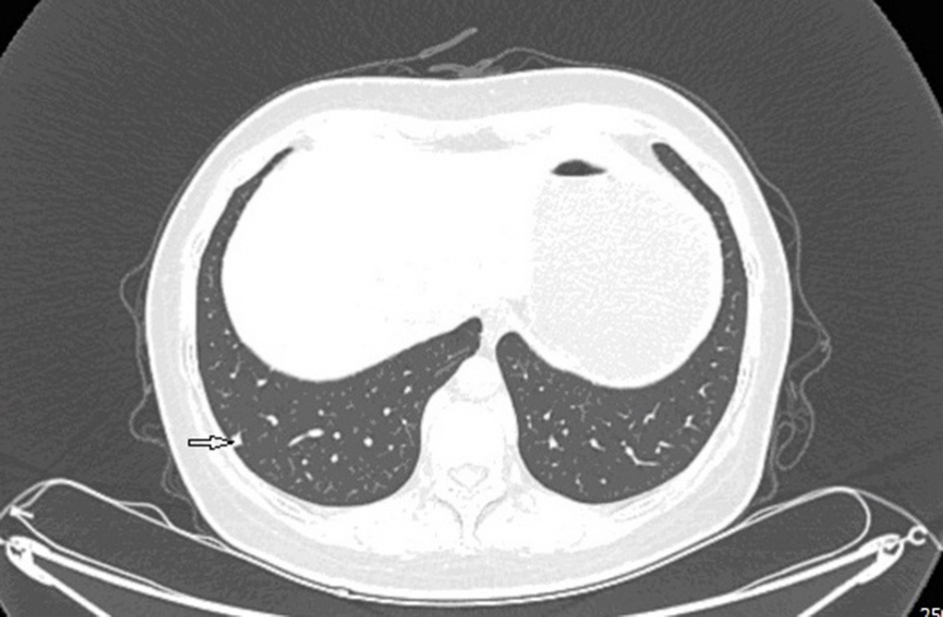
Contrast-enhanced CT images at 18 months after second surgery: lump in lower lobe of the right lung.
